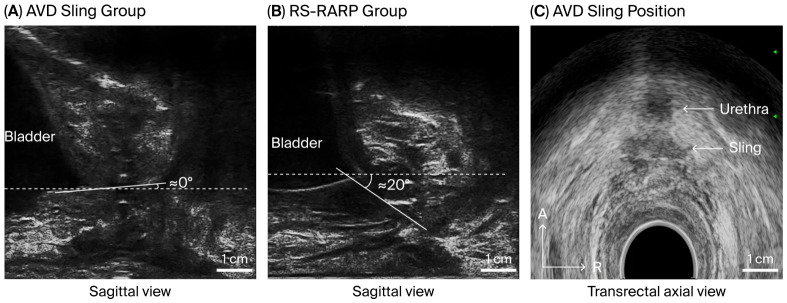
Representative postoperative imaging findings following RS-RARP with or without AVD sling suspension. (A,B) Comparison of the urethral inclination angle at resting status between the two groups. (A) In the AVD sling group, the proximal urethra demonstrates a nearly horizontal orientation. (B) In the RS-RARP group, the proximal urethra shows a more pronounced inclination angle. (C) A transrectal ultrasound image demonstrating the position of the autologous vas deferens sling beneath the vesicourethral anastomosis (the green triangles are part of the original ultrasound image generated by the ultrasound system).
